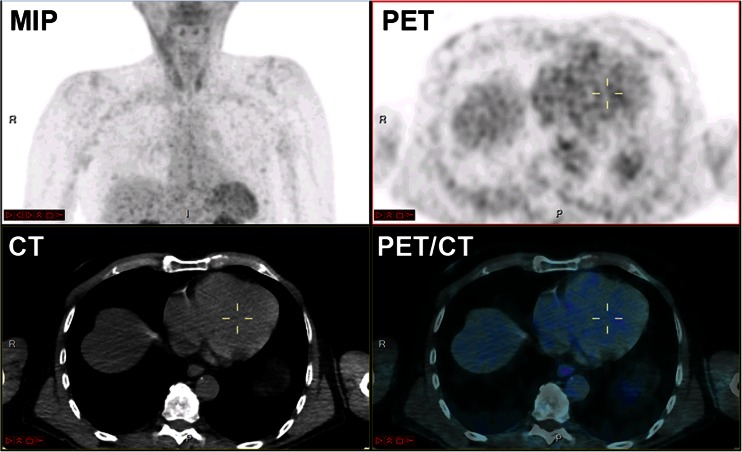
Example of Ga-68-NODAGA-RGD for imaging neoangiogenesis in a 72-year male patient with history of cardiac infarct 7 years prior to PET/CT imaging. Selected transaxial slice through the heart at 70-min post radiopharmaceutical injection showing no abnormal uptake in the old myocardial infarct indicating that no active angiogenesis is taking place
